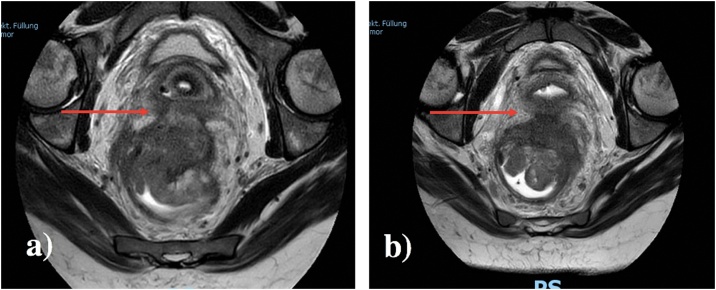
Pretreatment MRI: a) Large rectal tumour likely invading cervix (red arrow), b) rectal tumour invading posterior vaginal wall (red arrow).
